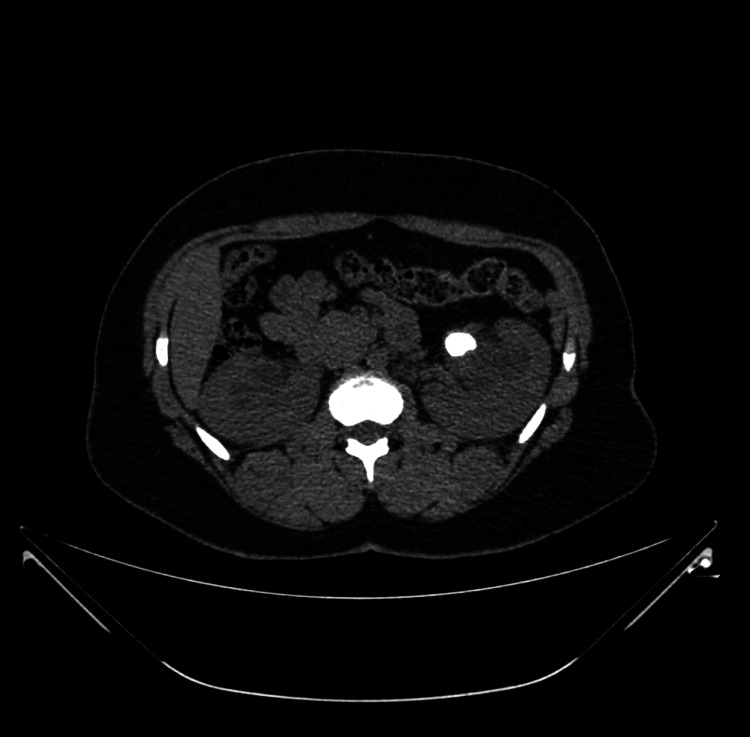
CT scan of abdomen - axial slice showing left renal pelvic stone with hydronephrosis
